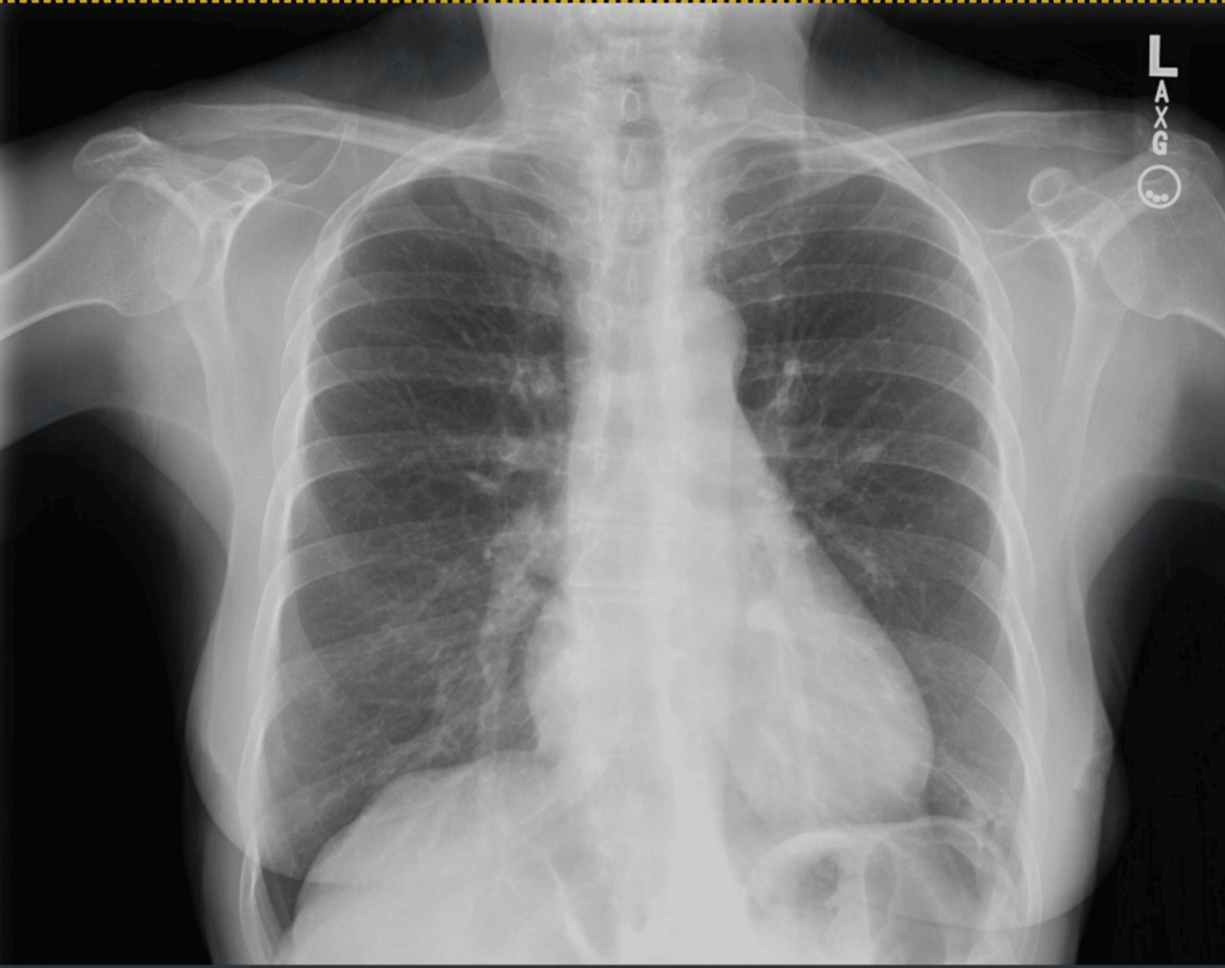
A chest X-ray without any significant findings to explain chronic cough.
